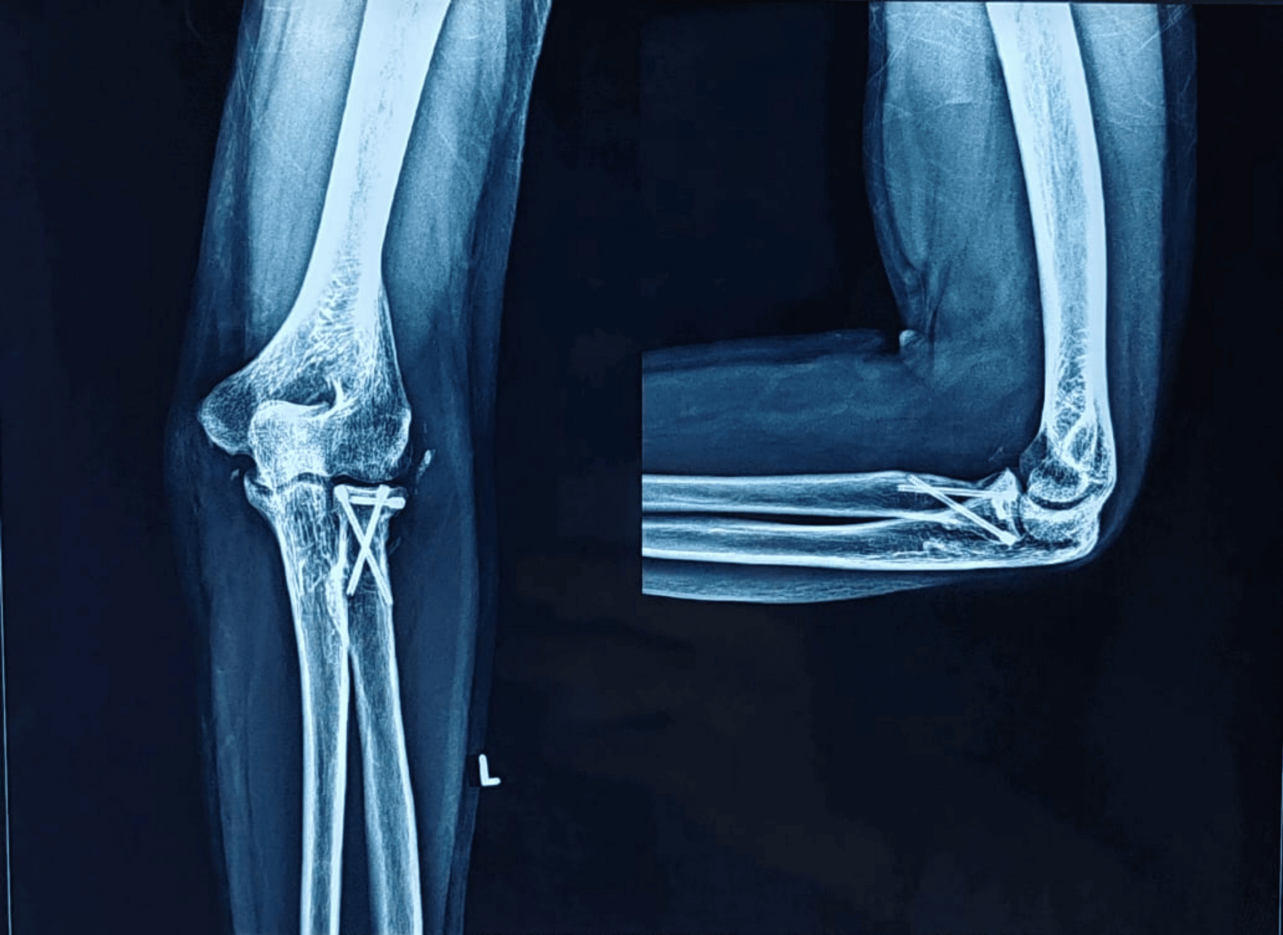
One-year follow-up X-ray depicting AP and lateral views of the left elbow joint AP: Anteroposterior
